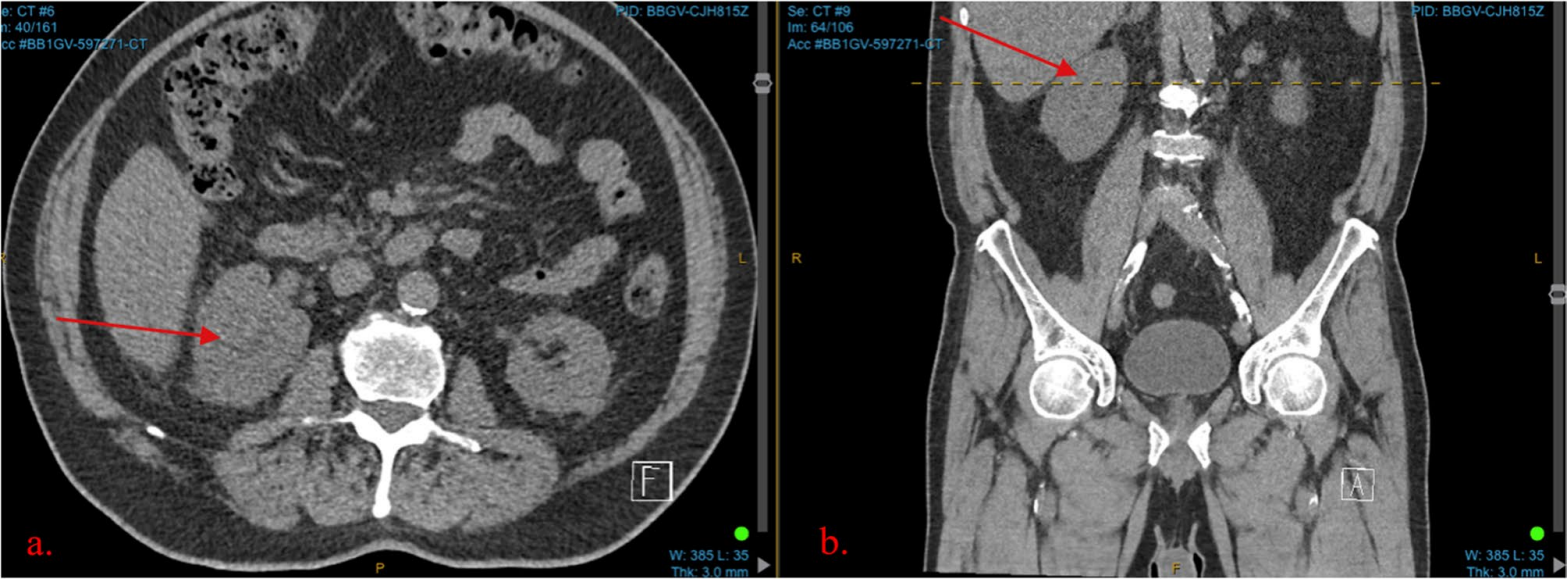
Non-contrast CT Kidney, ureter and bladder. a  An axial view demonstrating loss of clarity of right upper pole renal sinus. b A coronal view demonstrating the same
